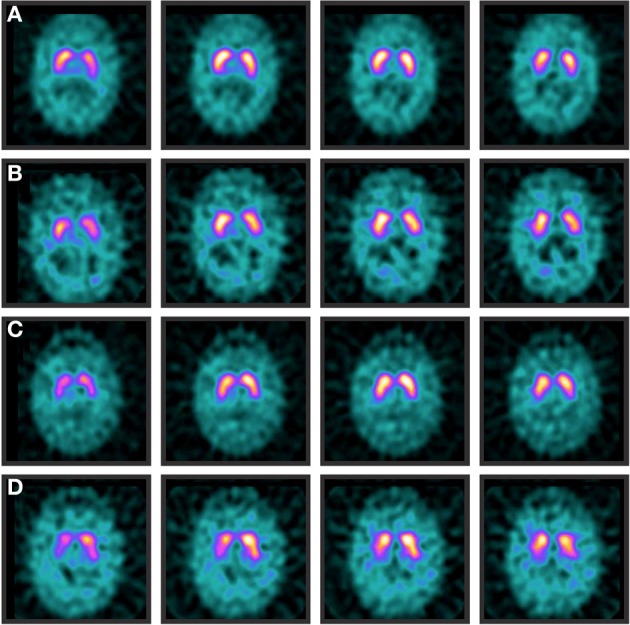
Normal and abnormal DAT-SPECTs. Normal DaT-SPECTs of a patient with essential tremor at baseline (A) and 42 months later (B). Normal DaT-SPECT of a patient with Parkinson's disease at baseline (C). However, 84 months later (D) the scan was abnormal due to a decrease in postsynaptic uptake on the right striatum.
